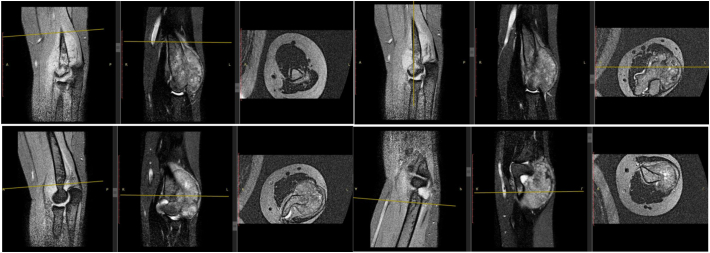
MRI examination of the left elbow showing low intensity signalling on T2 weighted.
